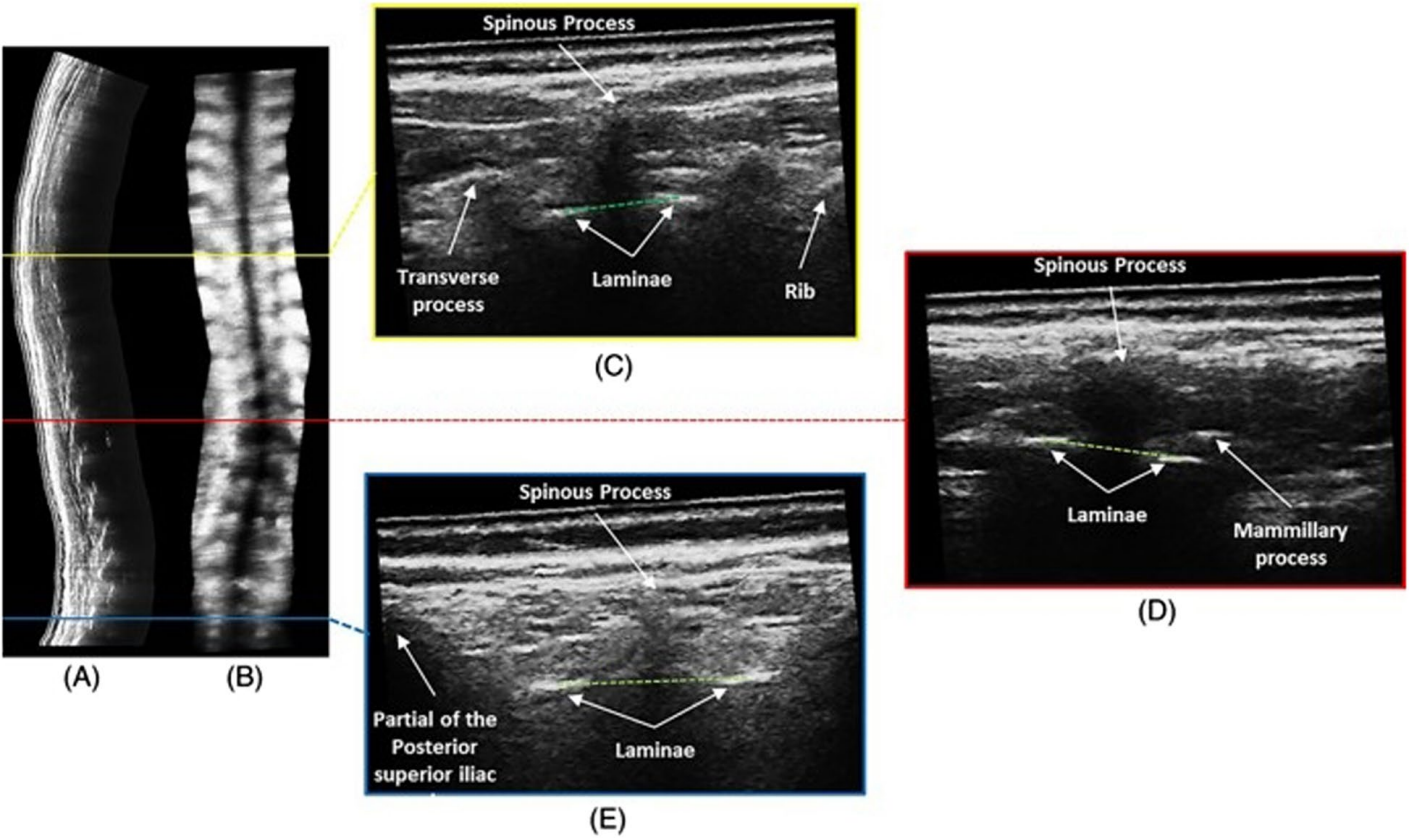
Center of lamina (COL) method for measurement of coronal curvatures and axial vertebral rotation on reconstructed 3D ultrasound images. Adopted with permission under Creative Commons Attribution License “Three-dimensional Ultrasonography Could be a Potential Non-ionizing Tool to Evaluate Vertebral Rotation of Subjects with Adolescent Idiopathic Scoliosis” by Lee et al. [23]. a Sagittal plane. bCoronal plane. Corresponding transverse plane at: c T8 level. d L1 level. e S1 level. The green dotted lines join the laminae at the 3 above mentioned levels
